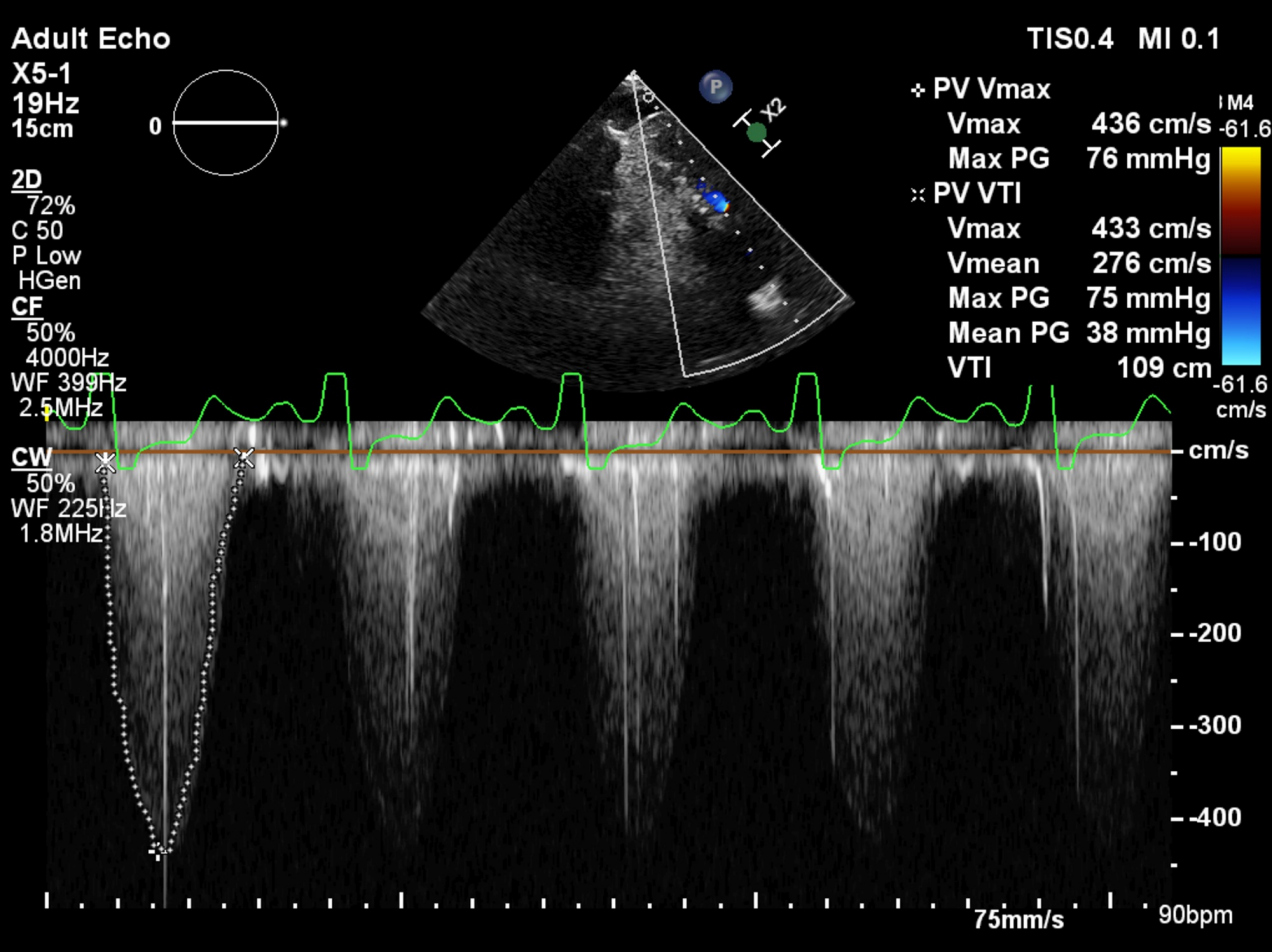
Transesophageal echocardiogram showing significant stenosis of the Melody valve
